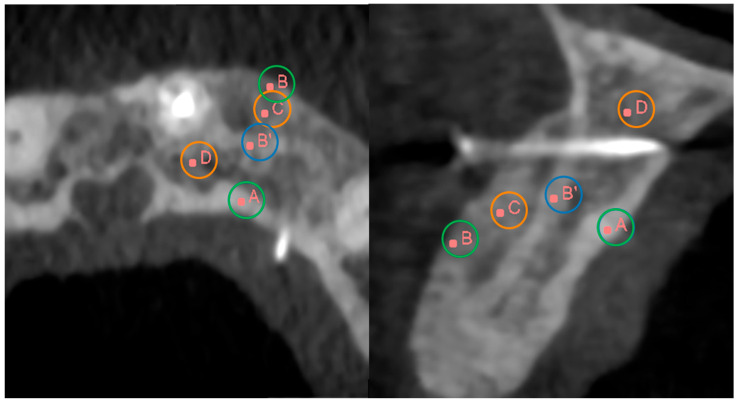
Axial (left) and sagittal (right) CBCT views of the maxillary bone. Scan images highlight key anatomical landmarks used to analyze bone regeneration after SmartBone^®^ grafting. Points of interest include: (A) lingual cortical, (B) new buccal cortical, (B’) original buccal cortical, (C) treated medullary, and (D) non-treated medullary. These points are used to calculate ΔCT values, assessing cortical and medullary remodeling over time.
